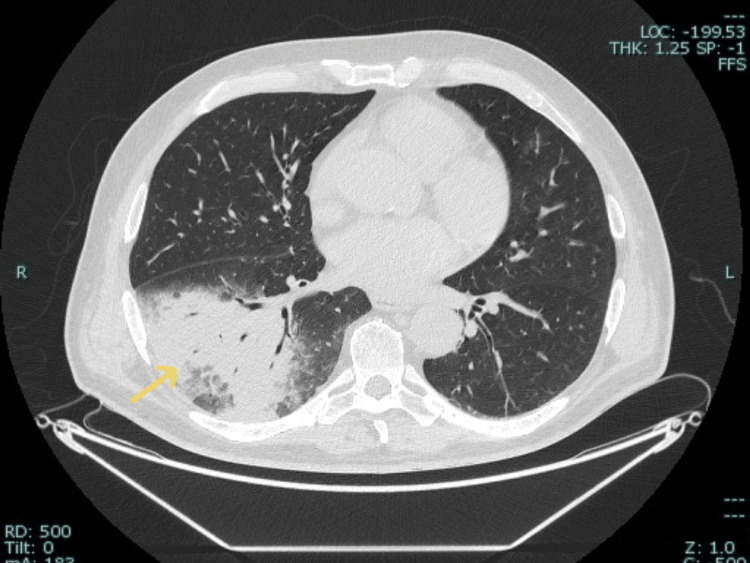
Non-contrast CT scan of the chest showing large consolidation in the right lower lobe, consistent with pneumonia (yellow arrow).
